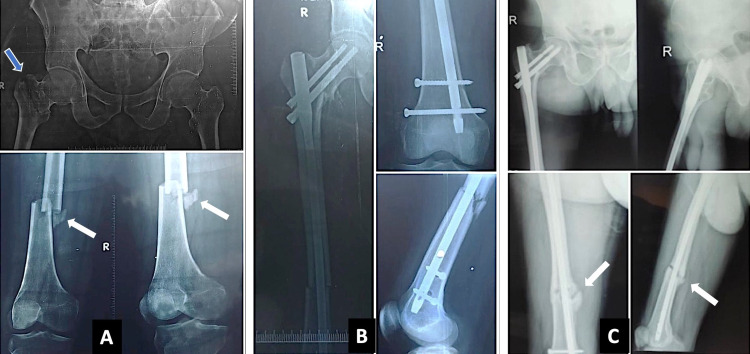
(A) Preoperative X-ray of one of the patients in our study showing intertrochanteric fracture (blue arrow) and infra-isthmic shaft fracture (white arrow). (B) Immediate postoperative X-ray of the patient after cephalomedullary nailing. (C) Follow-up X-ray at 12 months showing hypertrophic nonunion of the femoral shaft (white arrow).
